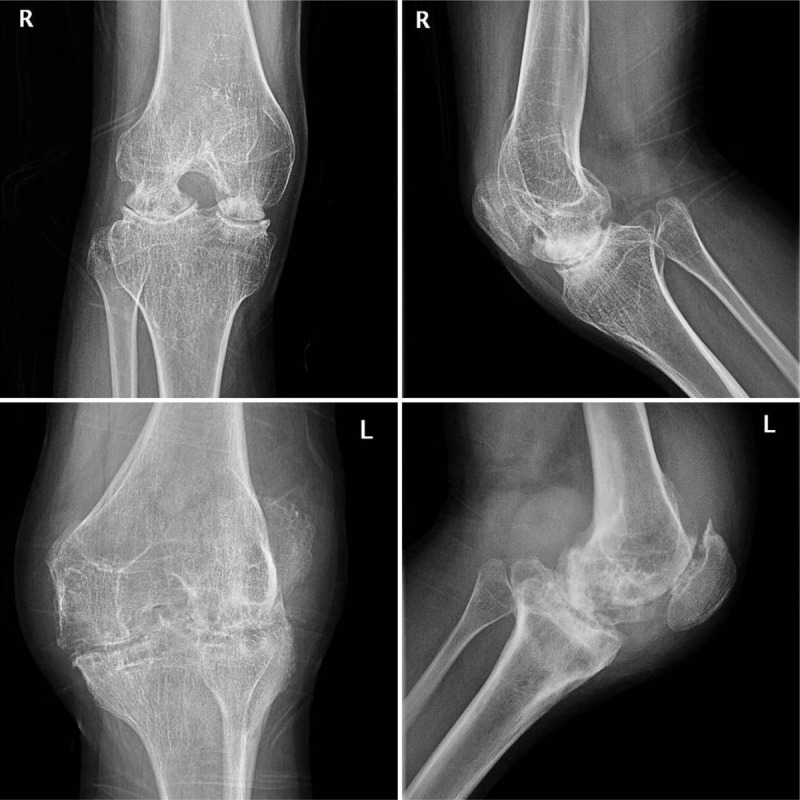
Radiography of a patient with hemophilic knee arthritis in both knees.
